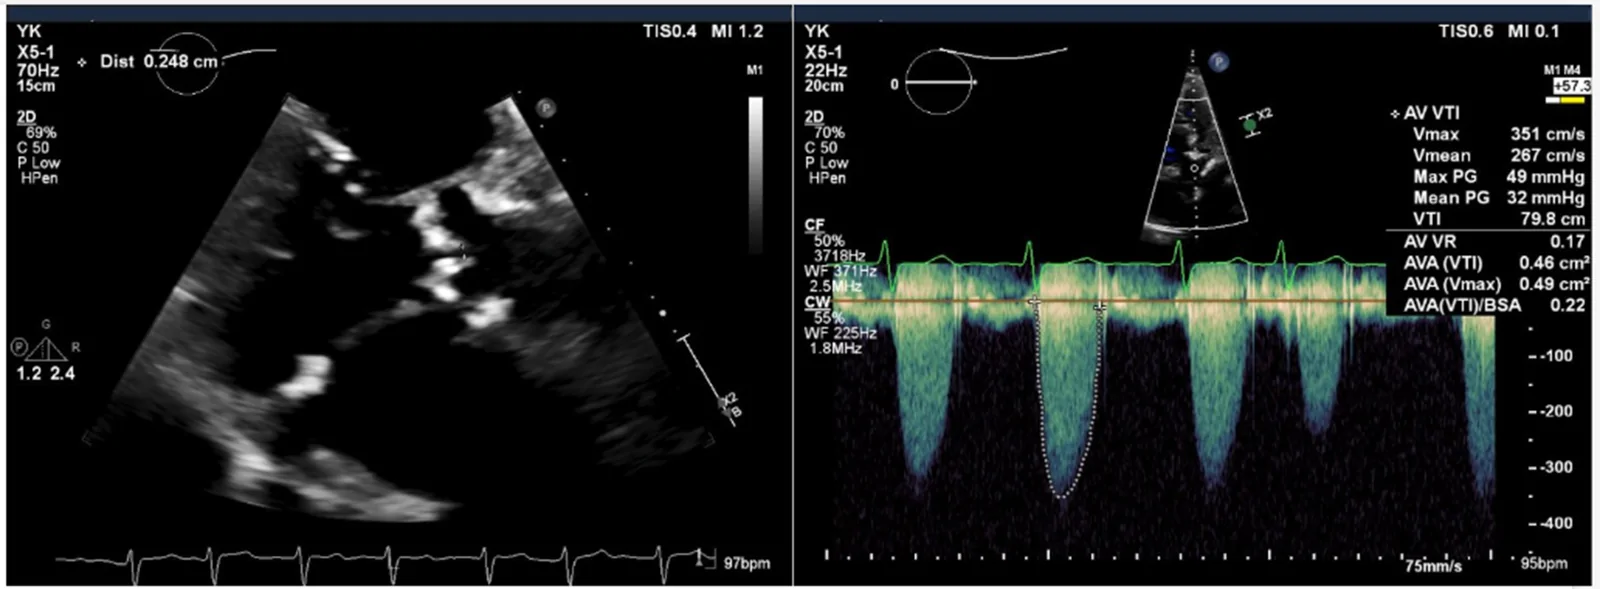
Transthoracic Echocardiography on Presentation Showing Aortic Stenosis (Left) Aortic-focused zoomed-in parasternal long-axis view demonstrating severely reduced aortic valve cusp separation (0.25 cm). (Right) Continuous-wave Doppler in the apical 4-chamber view demonstrating aortic valve area of 0.49 cm^2^, suggesting critical aortic stenosis, with a mean gradient of 32 mm Hg, and maximum velocity of 3.51 m/s.
